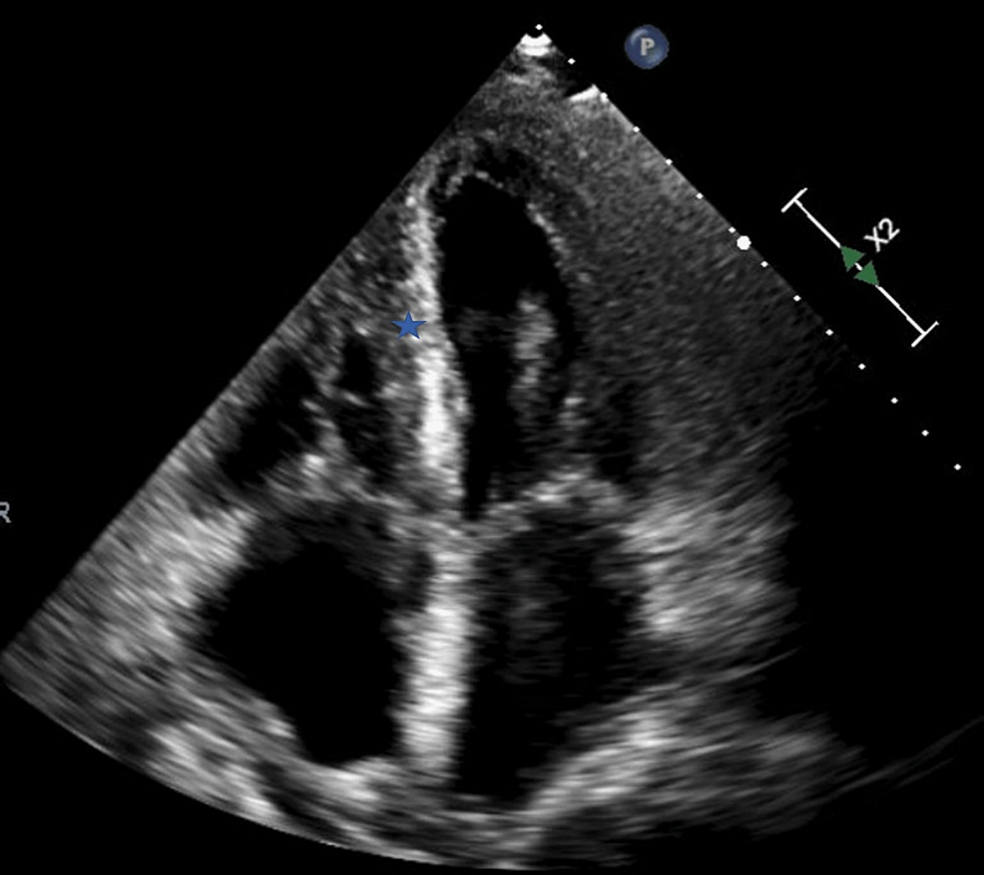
Echocardiogram Apical four-chamber view showing increased left ventricular septal wall thickness (blue star) and bi-atrial enlargement. The ejection fraction here was 27%, and there was severe diastolic dysfunction
